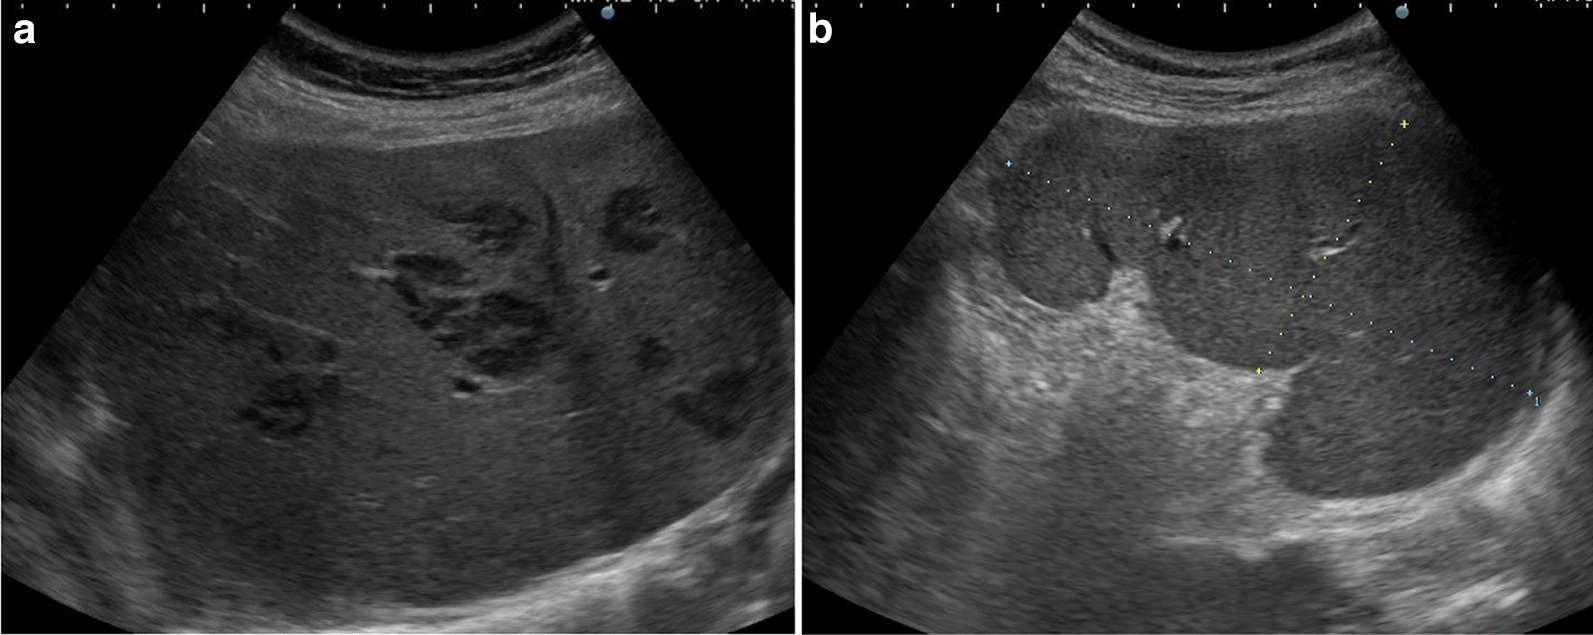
Ultrasound findings. a Multiple well-defined hypoechoic hepatic nodules, b splenomegaly without focal lesions
